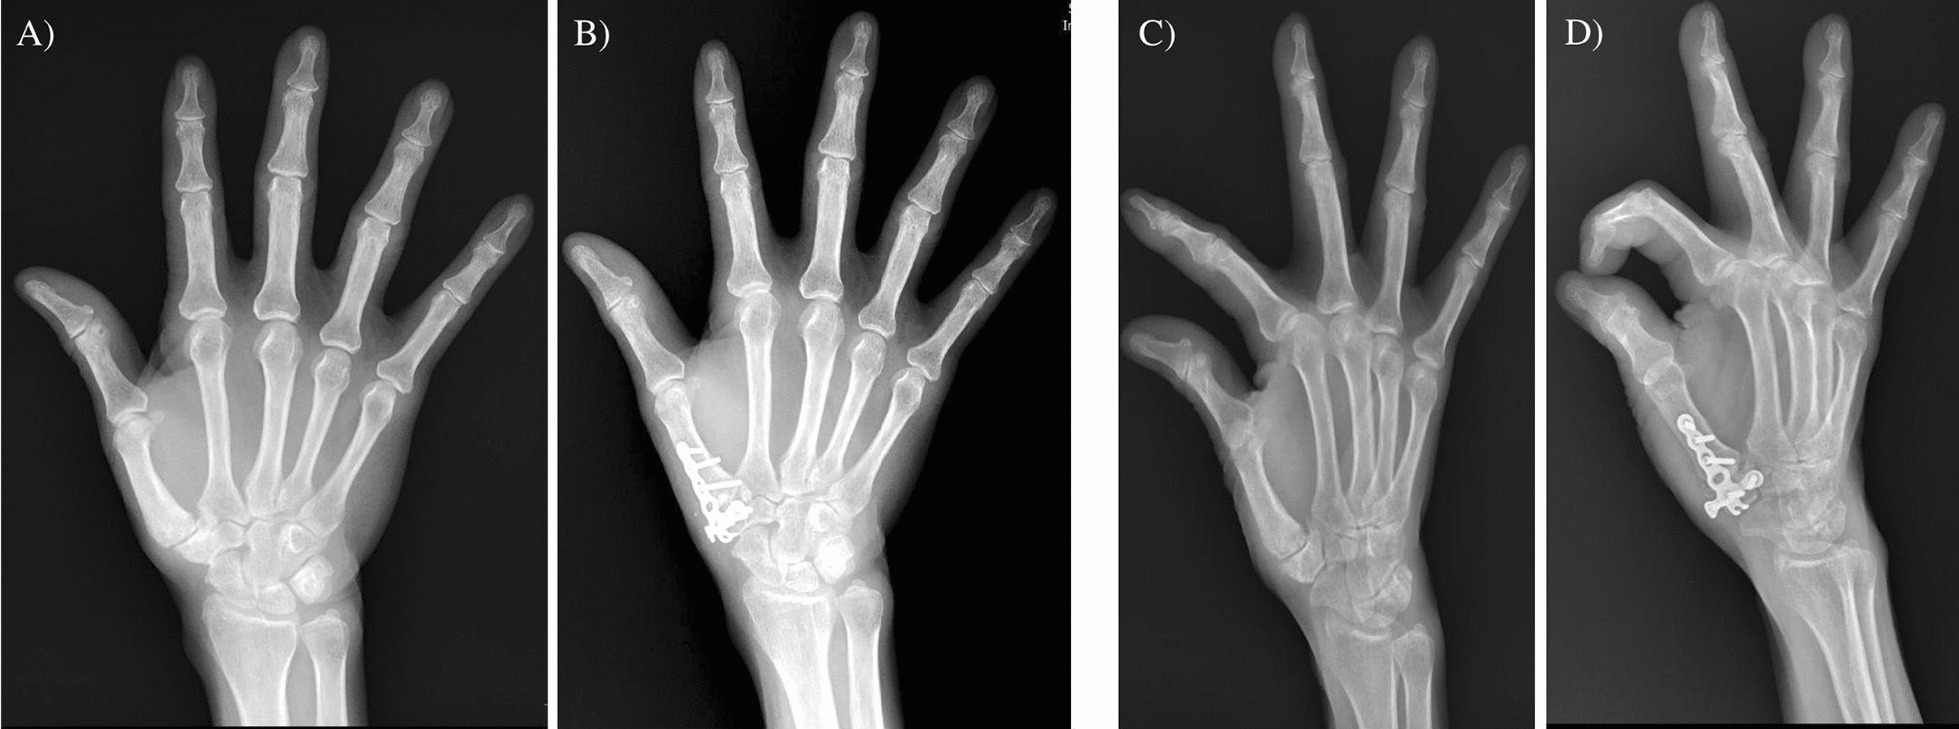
Hand X-rays A AP and C oblique preoperative X-rays; B AP and D oblique postoperative X-rays with T-hook plate CMC joint fusion
